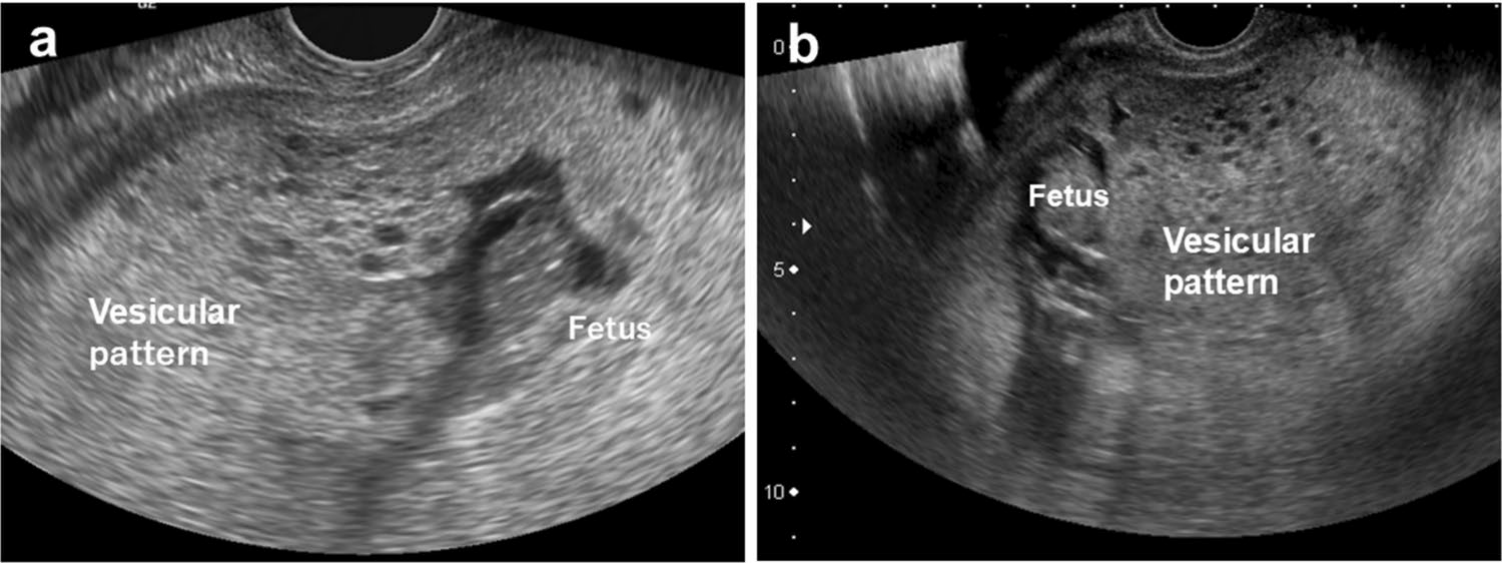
Ultrasound findings. Ultrasound images of cases 2 (a) and 9 (b), revealing a cystic mole and a portion of the fetus in both cases
